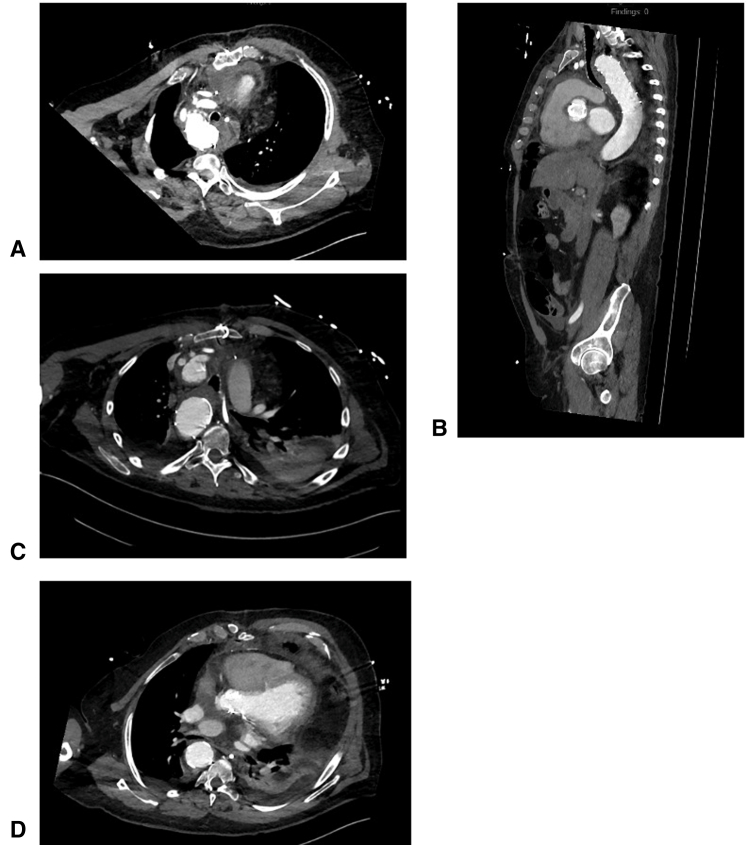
Post-frozen elephant trunk computed tomography demonstrating persistent airway compression. Axial (A, C-D) and sagittal (B) computed tomography showing a thrombosed Kommerell diverticulum located between the frozen elephant trunk and the occluded left subclavian artery with absence of endoleak. There was persistent severe compression of the distal trachea and right main bronchus.
